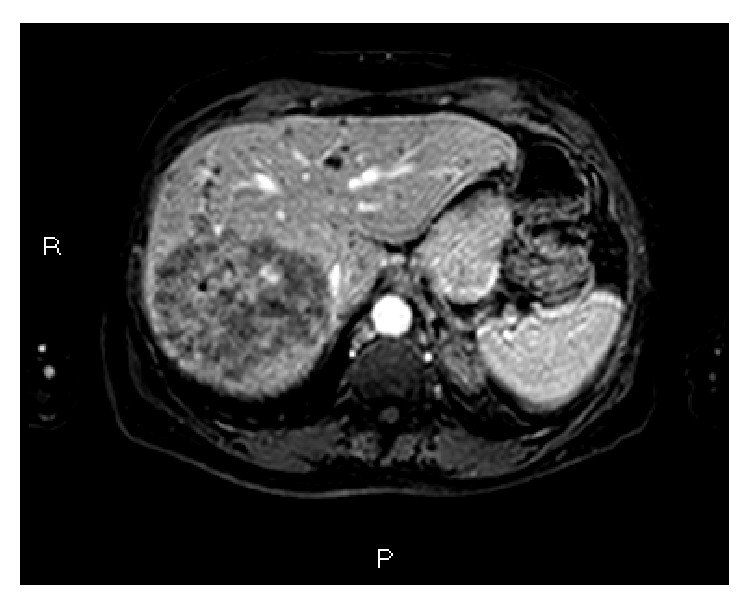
Gadolinium enhanced T1 weighted MRI of the abdomen with fat saturation prior to treatment with radioembolization shows right hepatic lobe with 8 cm × 9 cm mass (06/07/2013). Enhancement within the tumor indicates tumor viability.
